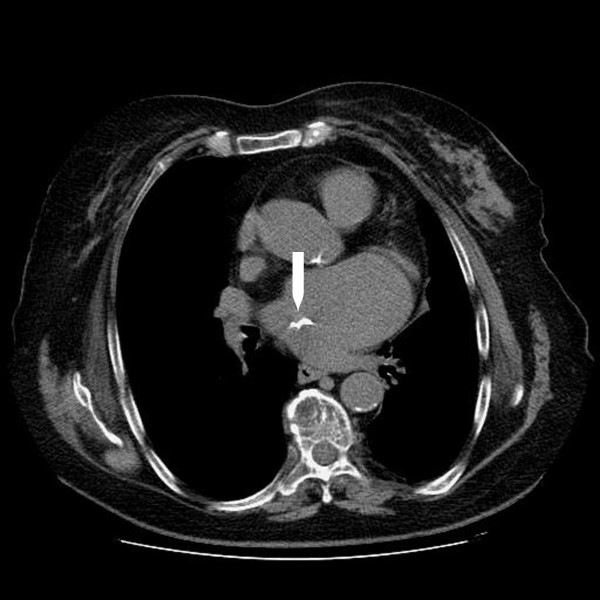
Detection by computed tomography of lipomatous hypertrophy of the interatrial septum.
